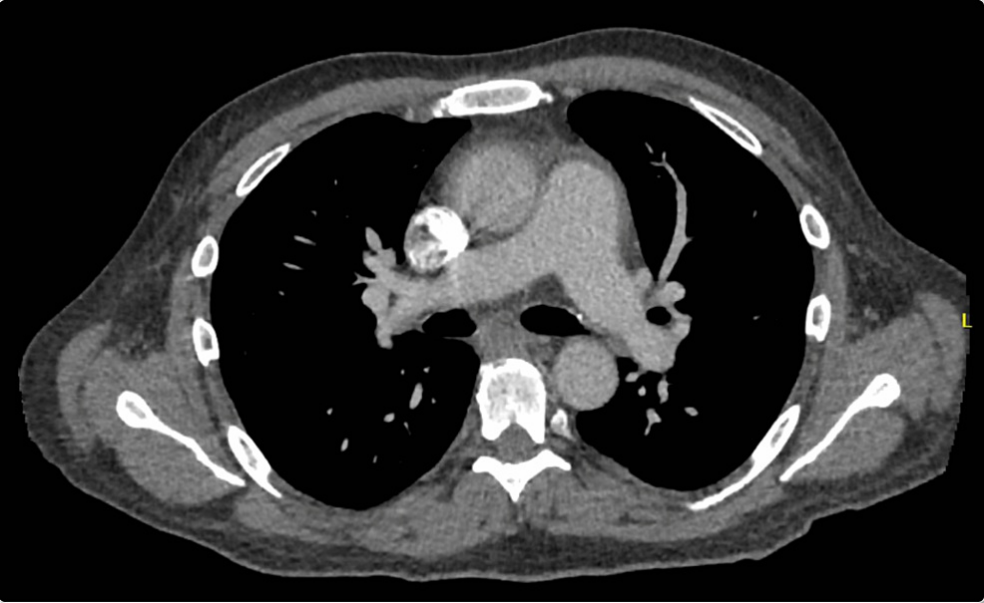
Initial CT Angiography Mild pericardial effusion surrounding the heart as seen in the increased thickness of the pericardial border with fluid-density attenuation.
